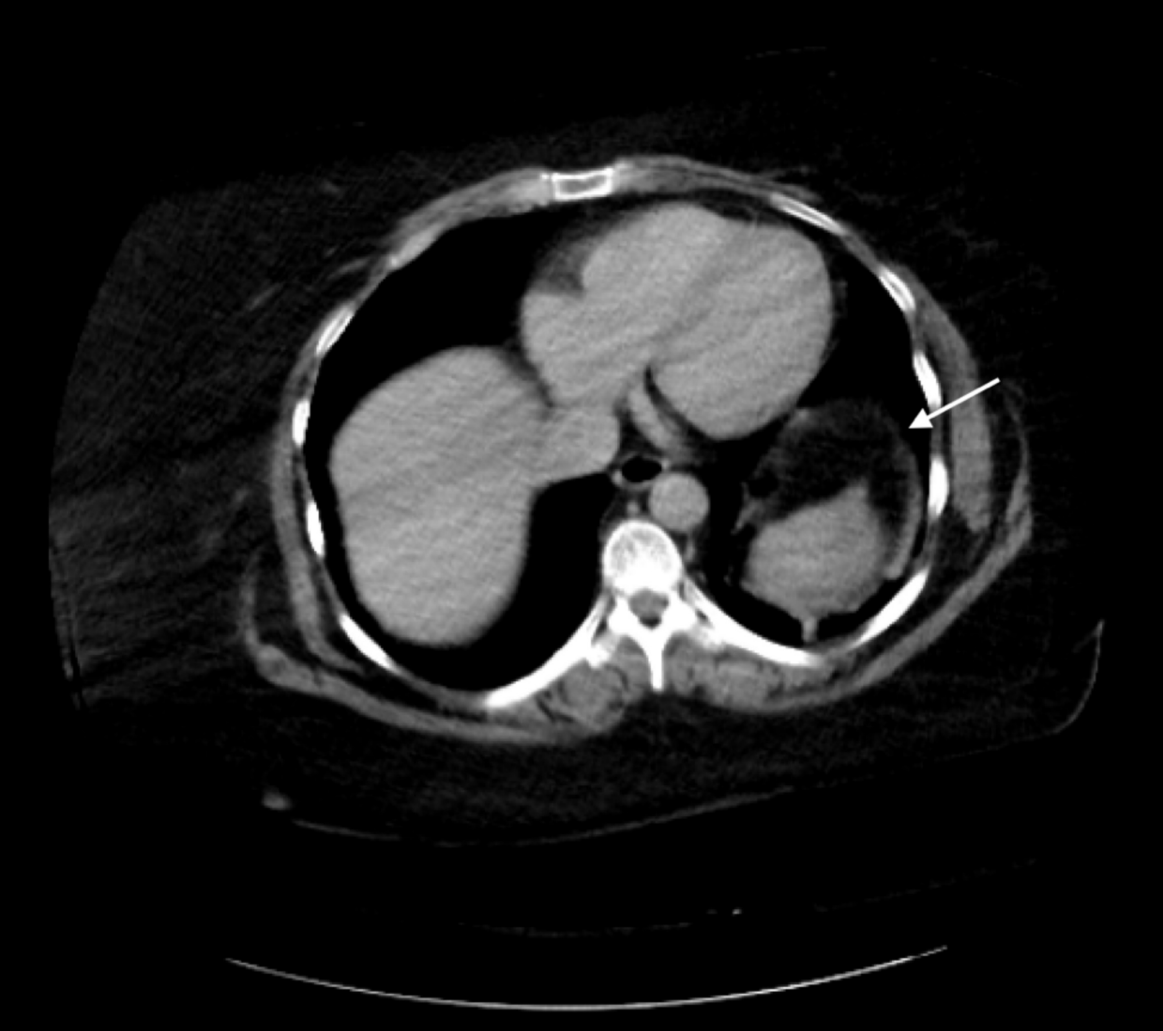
CT abdomen and pelvis consistent with large grade 3 splenic laceration and hematoma (white arrow)
